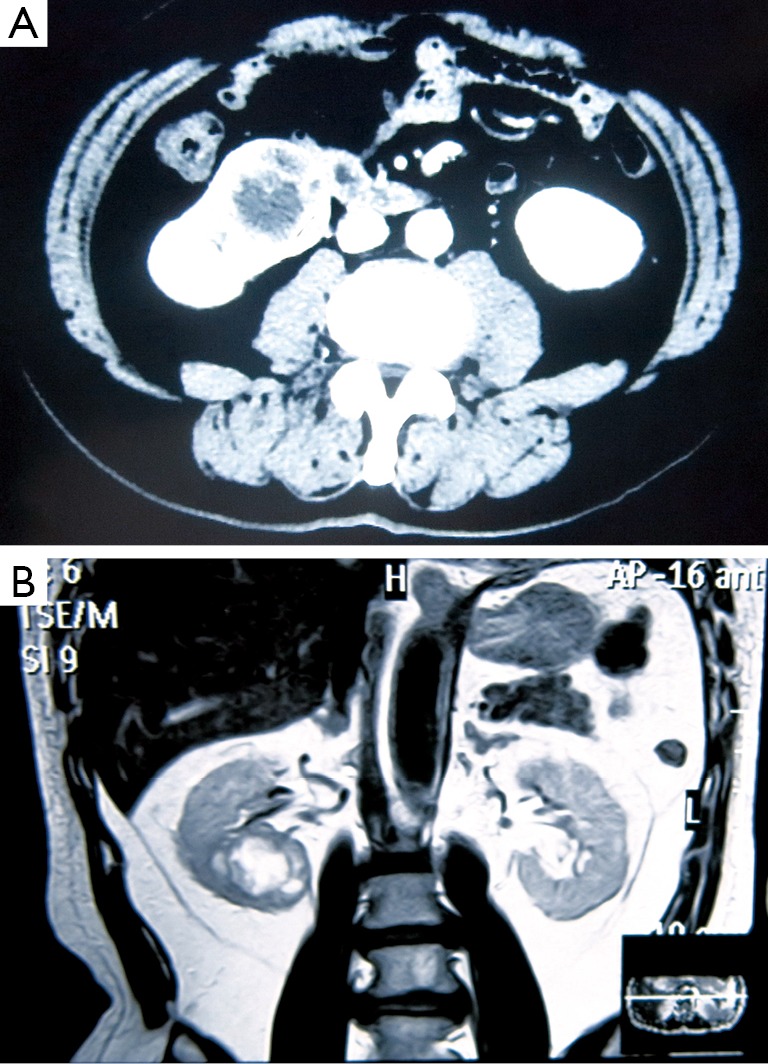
A tumor in the right renal cortex, spreading to the renal capsule. (A) CT. (B) MRI.
